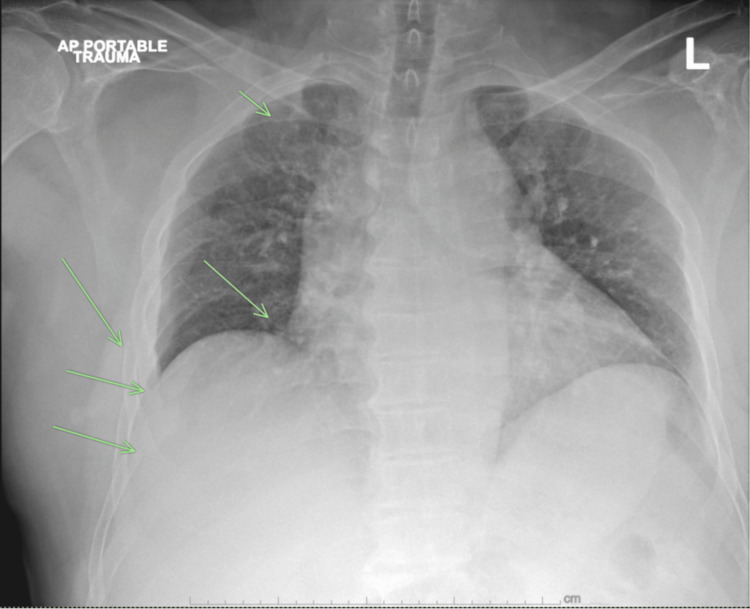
Chest X-ray demonstrating multiple right rib fractures (green arrows) and mediastinal widening.
